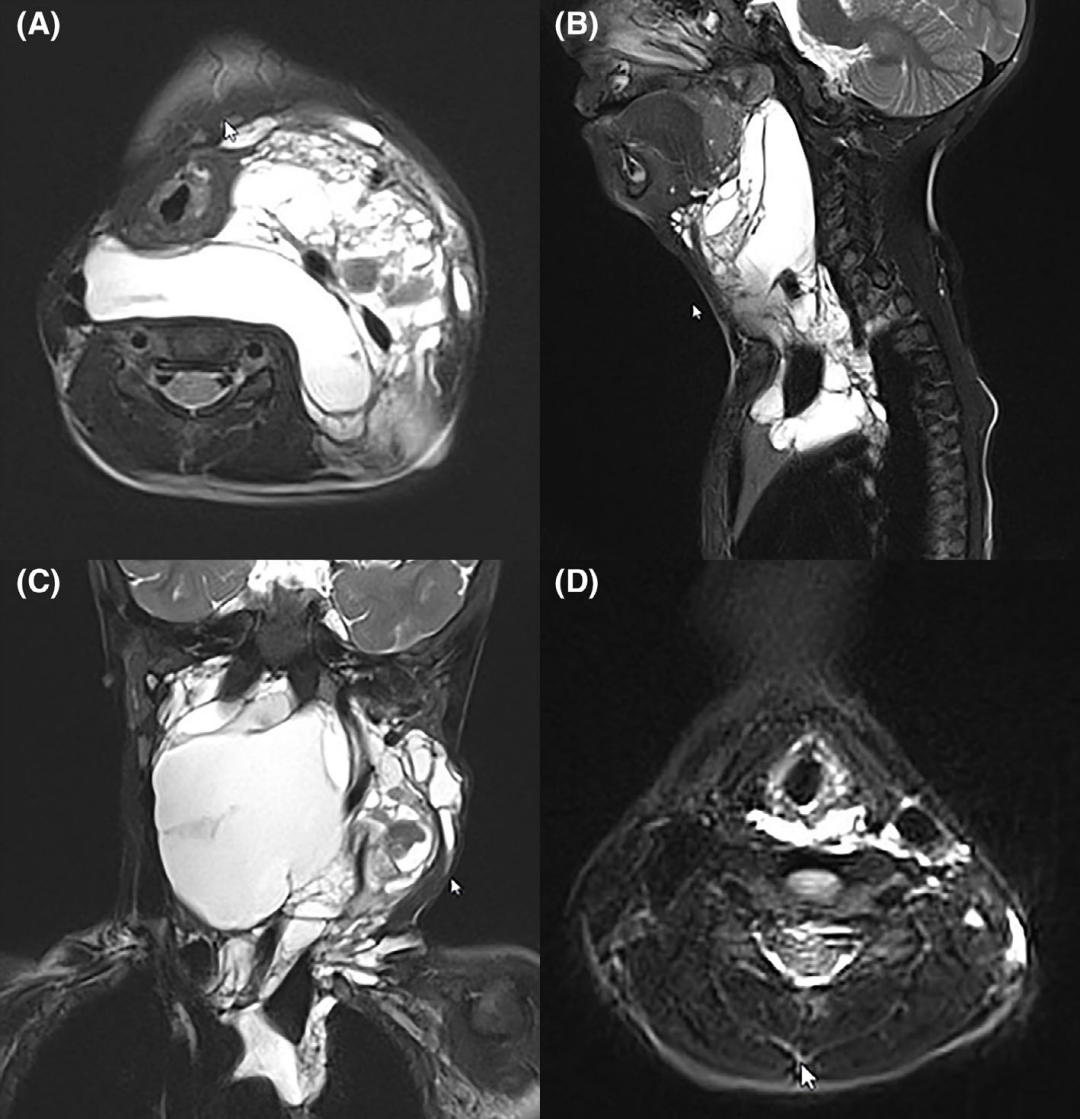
Same child as in Figure 3. A (axial), B (sagittal) and C (coronal) preoperative MRI at 2 years of age. The mixed lymphatic malformation insinuates between cervical plans and causing moderate–severe degree of extrinsic airway obstruction and mediastinal extension. (D) Axial MRI at 7 years of age, following surgery and intralesional sclerotherapy with Bleomycin
